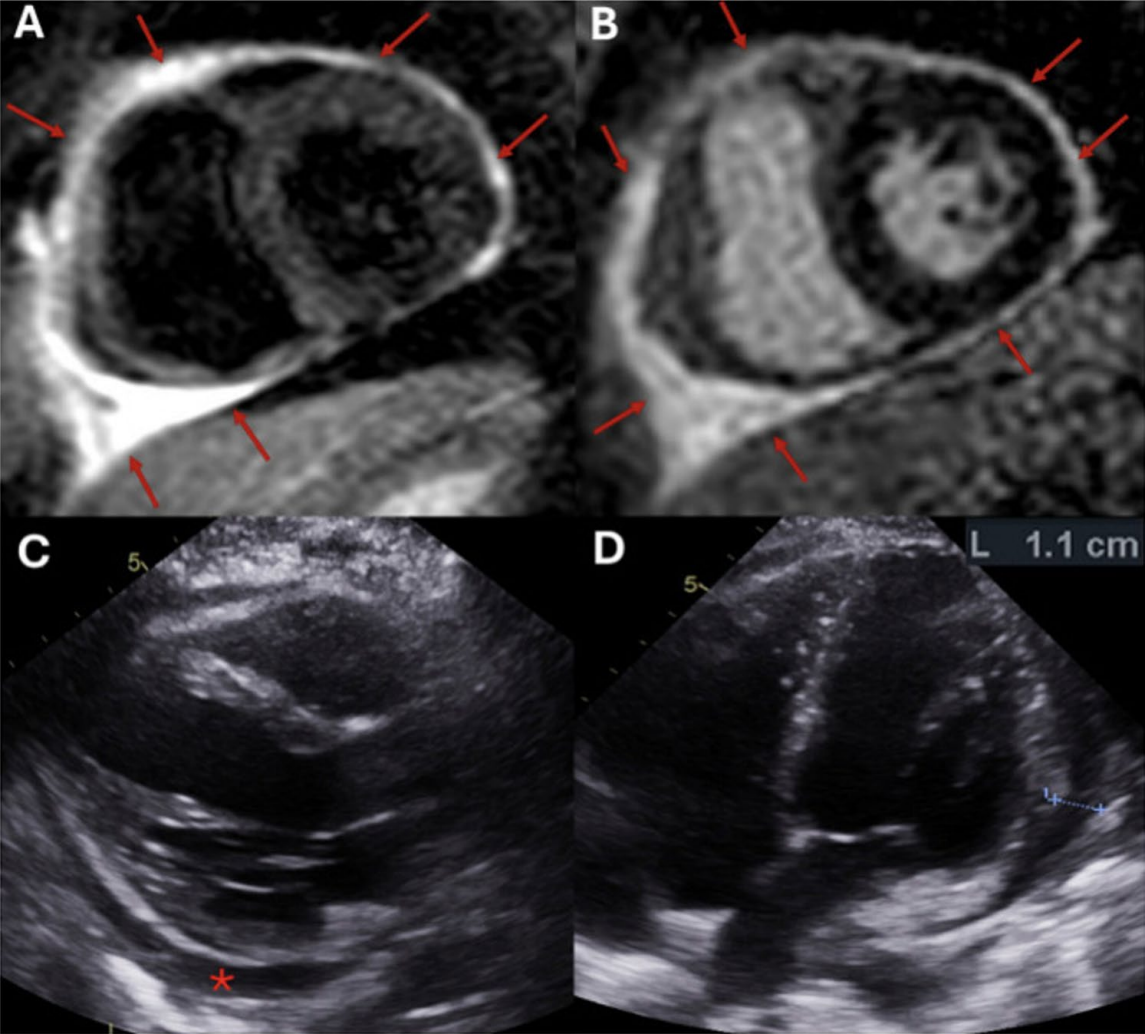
Multimodality imaging findings in active pericarditis with pericardial effusion. Panel A: Cardiac MRI T2-STIR sequence showing marked circumferential pericardial hyperintensity consistent with inflammation and edema (red arrows). Panel B: Cardiac MRI delayed enhancement sequence demonstration circumferential pericardial late gadolinium enhancement (LGE), indicating active inflammation and fibrosis (red arrows). Panel C: Transthoracic echocardiogram (parasternal long-axix view) showing a small circumferential pericardial effusion (asterisk). Panel D: Transthoracic echocardiogram (apical four-chamber view) demonstrating pericardial effusion measuring 1.1 cm lateral to the left ventricle (asterisk)
